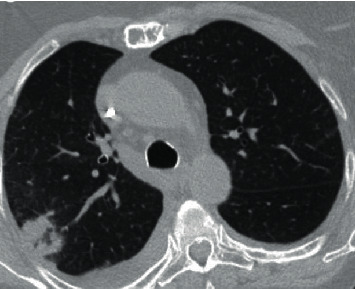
Initial chest CT showing right lobe infiltrate.
